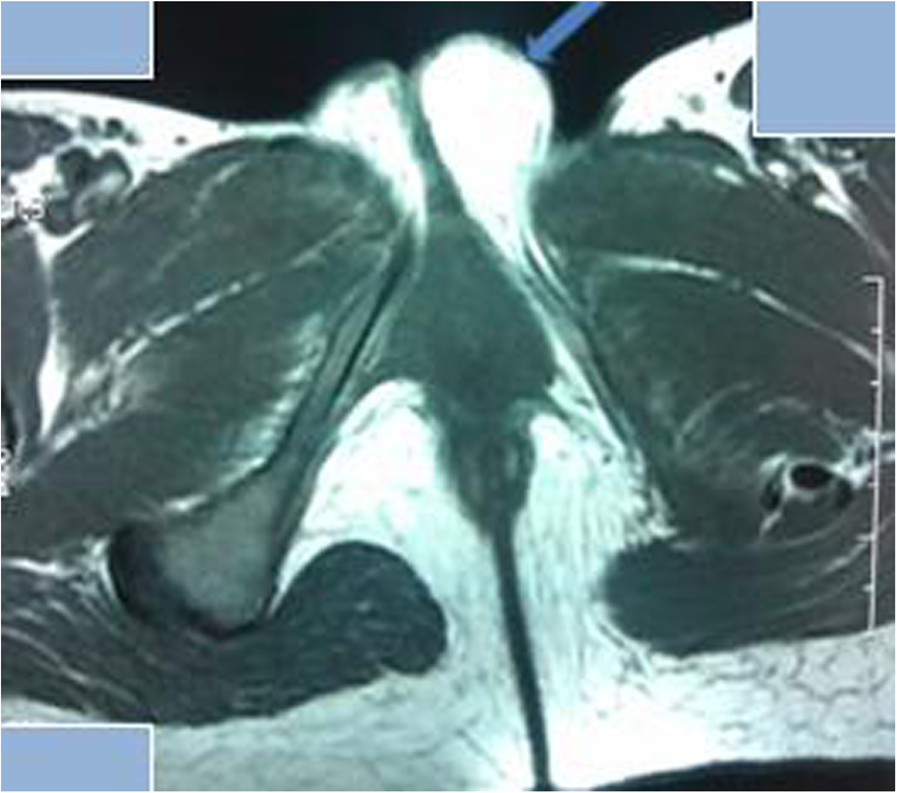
Axial T1-weighted magnetic resonance image shows a homogenous, hyperintense mass with a well-defined margin in the left labium majus (arrow).
